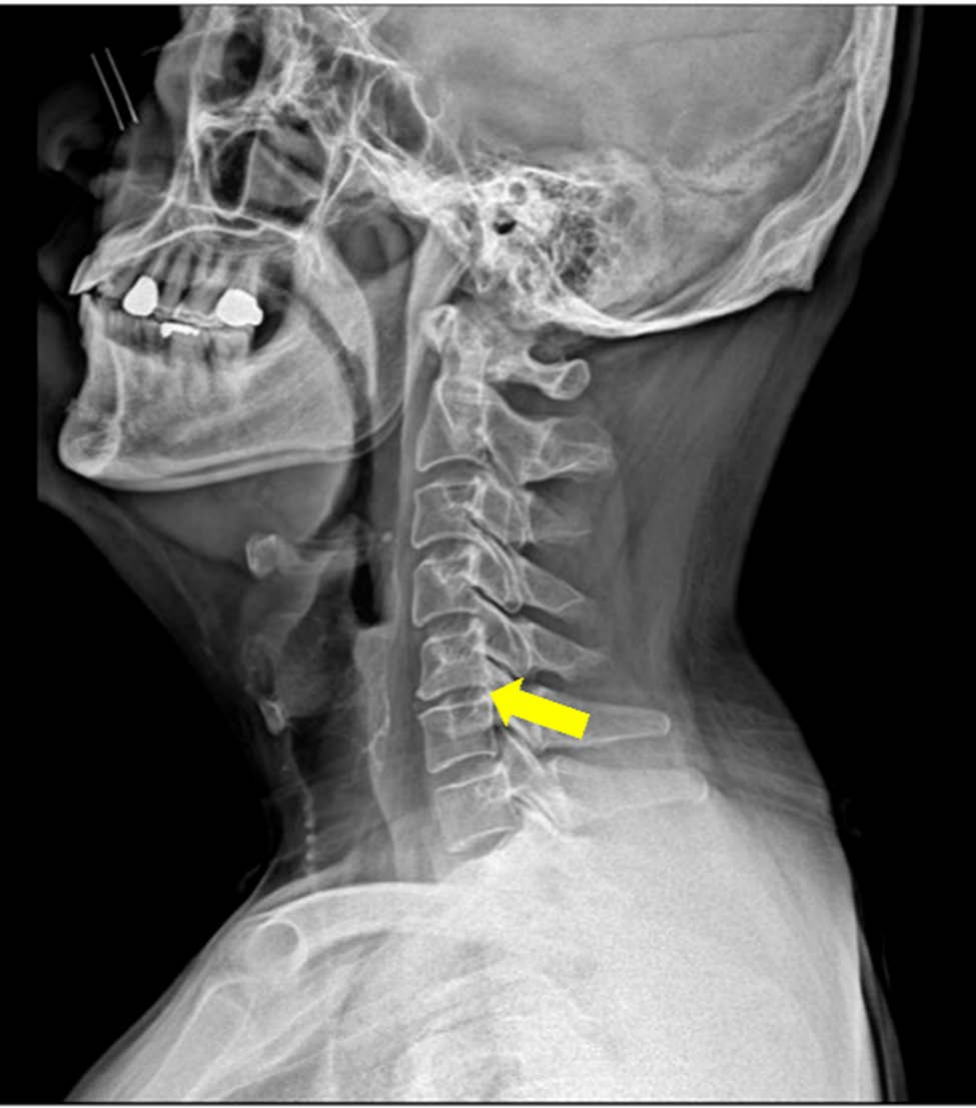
C-spine X-ray (October 27, 2023).
